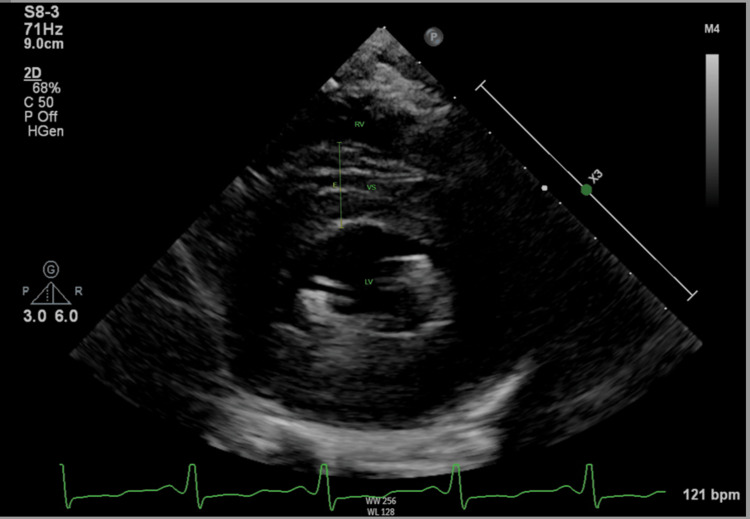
Echocardiography short-axis view showed septal hypertrophy at mid-basal, measuring 17.2 mm, during the systolic phase. RV: right ventricular; VS: ventricular septum; LV: left ventricular.
